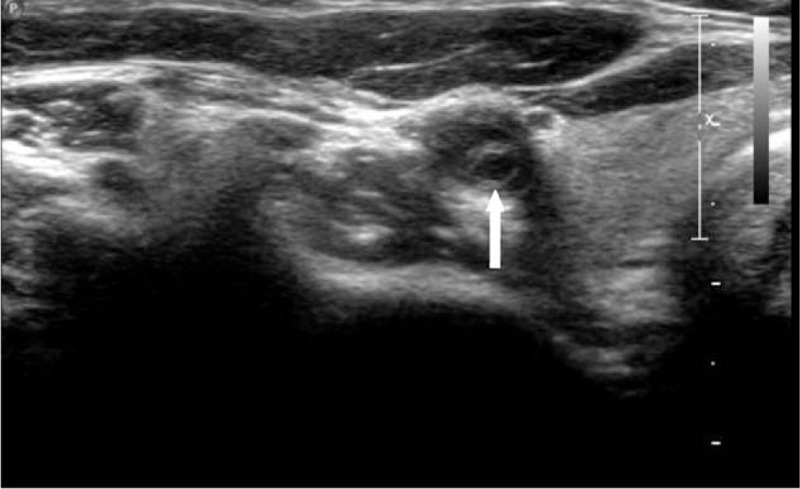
Transverse section of color Doppler ultrasound scan demonstrated the typical “fish mouth” (arrow) shape of diaphgram.
